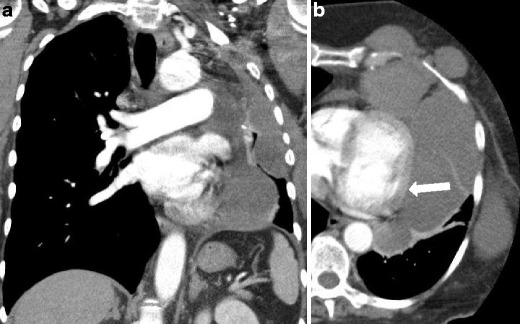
Large left thoracic wall sarcoma; contrast-enhanced CT scan: coronal (a) and axial (b) view. Severe left hemithorax hypoexpansion, homolateral hemidiaphragm superelevation and presence of a large polylobulated mass, with faint enhancement and extraparietal extension. No precise fat plane may be seen between the mediastinal pleura and the pericardium (*white arrow*), a finding that is highly suspicious for mediastinal infiltration
